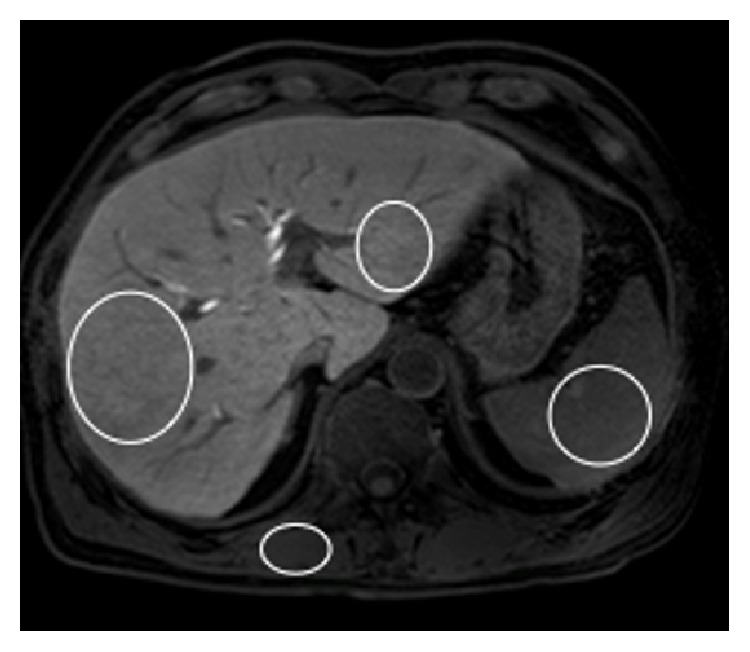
Hepatobiliary phase of Gd-EOB-DTPA-enhanced MRI. The signal intensities were measured by placing the largest possible regions of interest (ROIs) on the liver parenchyma, spleen, and erector spinae muscle, avoiding vessels, tumors, and artifacts. For the liver parenchyma, two round or oval ROIs were placed: one in the right lobe and the other in the left.
